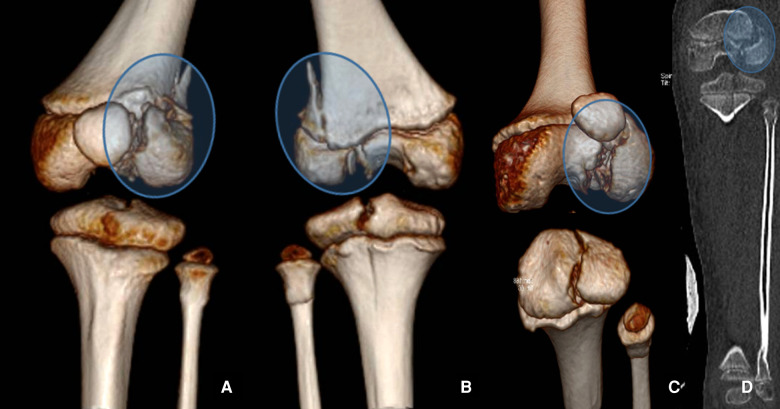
3D CT reconstruction of physis injuries of the left knee in anterior view (A), in posterior view (B), inlet view (C), and 2D CT coronal view (D).
